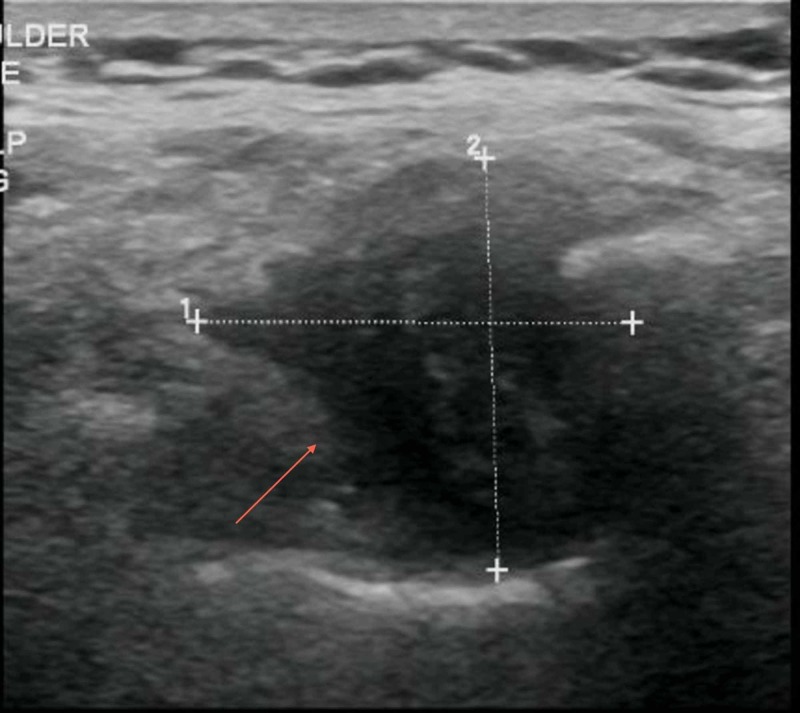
Ultrasound of right periscapular region showing a complex hypo-echoic mass
